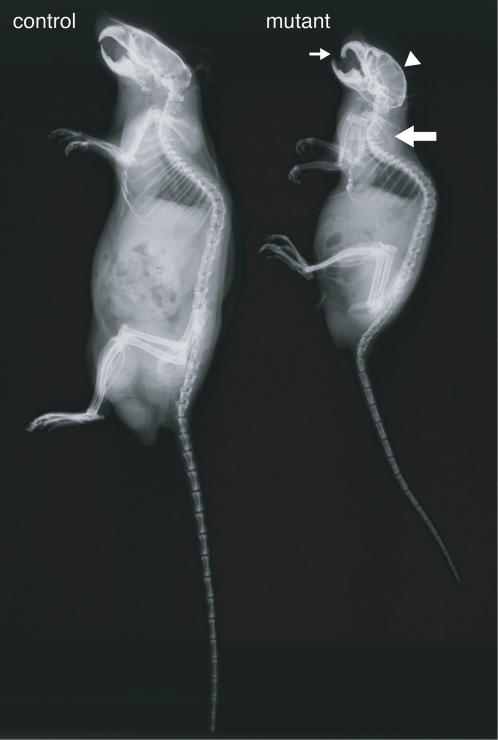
Radiographic analysis for 9-month-old *R26^floxneoWnt4^*
